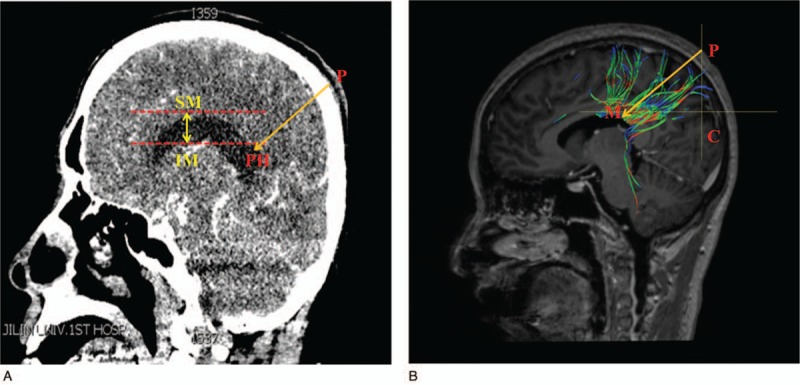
(A) The data and an innovative puncture method from the parietal lobe in computed tomography image. (B) The new innovative method's puncture point and angle in diffusion tensor image.
